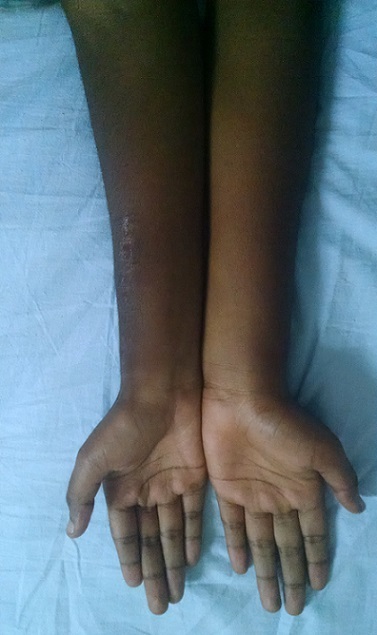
Radiographie de contrôle à la consolidation à 06 mois post opératoire
